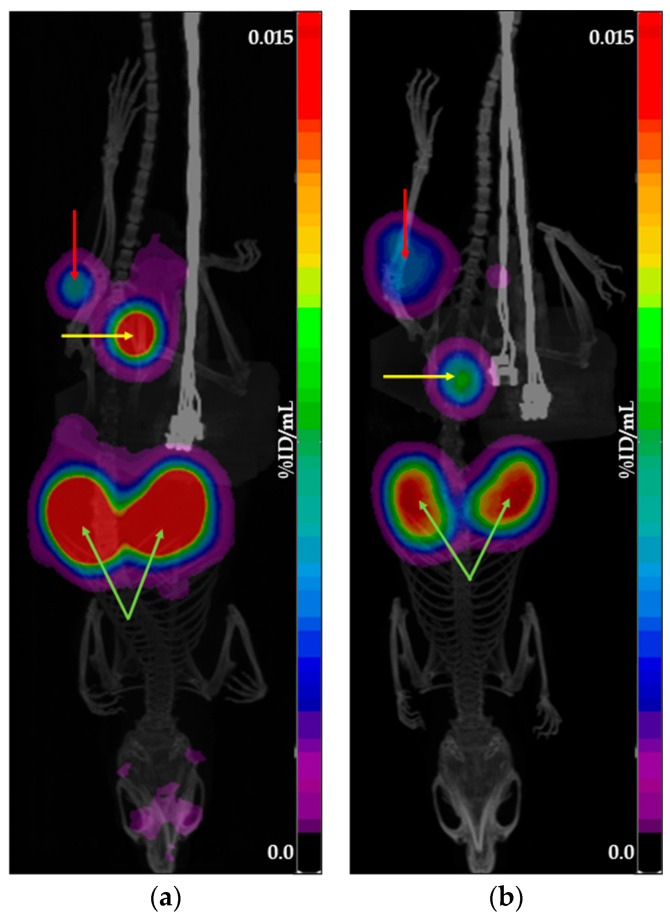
Example of PET/CT scans obtained using [^68^Ga]Ga-PSMA-11: (a) produced with cyclotron solid target isotope; (b) produced with generator-origin isotope. The images show the main places of tracer accumulation: the red arrow indicates the tumor, the yellow arrow indicates the bladder, and the green arrow indicates the kidneys.
